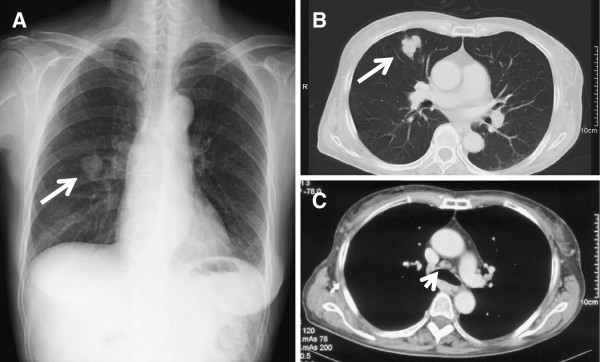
Chest radiograph and computed tomography scan at diagnosis. (A) Chest radiograph showing a mass shadow in the right middle zone (arrow). (B, C) Selected sections of a conventional computed tomography scan of the chest showing a 30-mm solitary mass in S^5^ of the right lung (arrow), and mediastinal lymphadenopathy (arrowhead).
